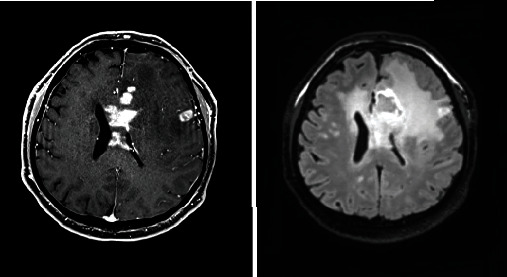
T2/FLAIR hyperintense lesion with patchy enhancement in the genu of the corpus callosum. Radiographic differential diagnosis: demyelinating lesion like ADEM and glioblastoma.
